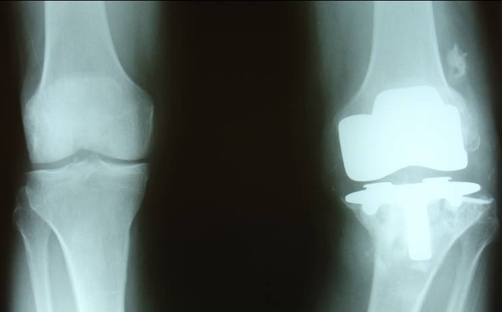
AP x-ray after 5 years of primary left knee arthroplasty showing premature aseptic failure.
